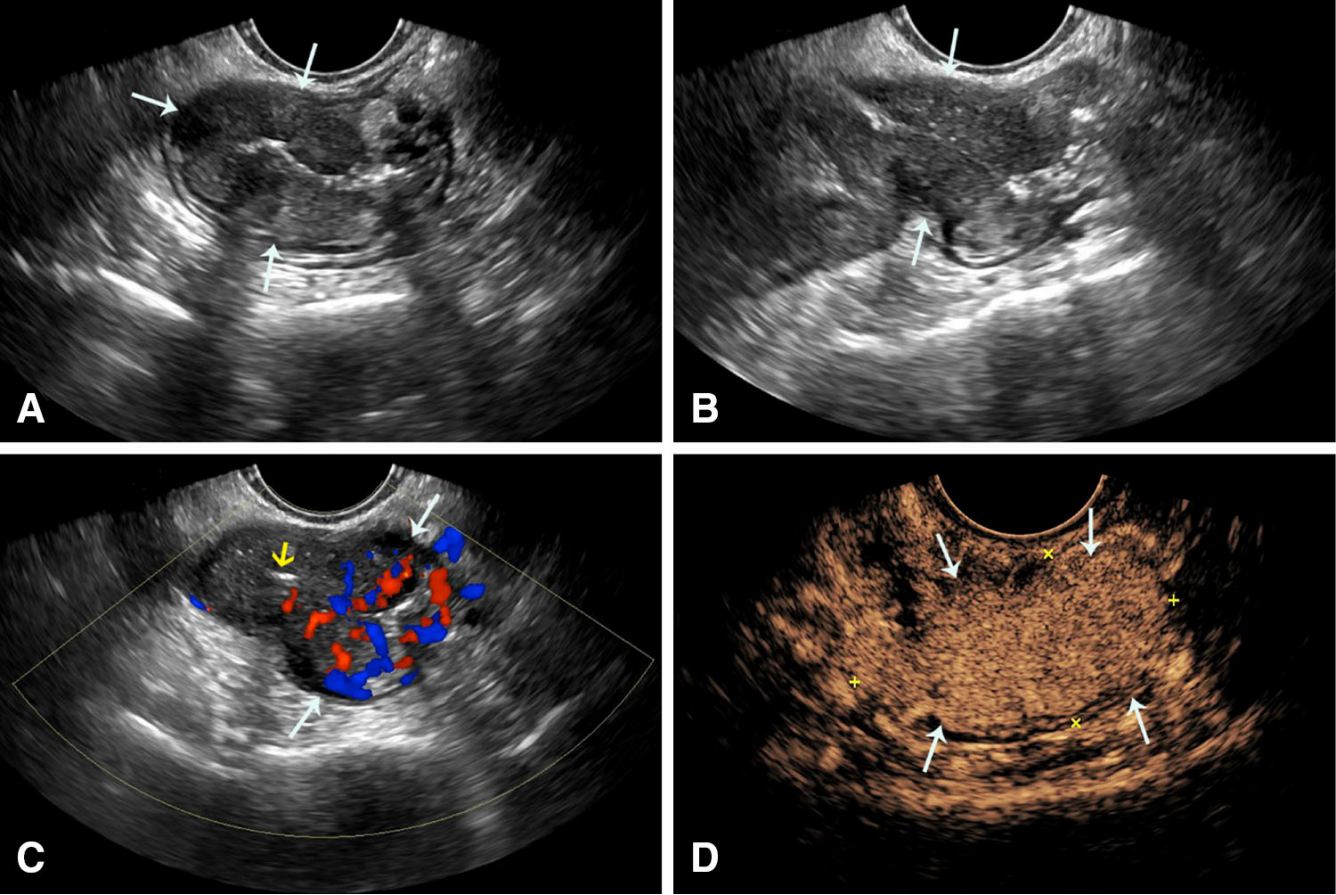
Rectal cancer on Endovaginal scan (EV) on a 37-year-old patient with rectal bleeding. A EV grayscale shows a lobulated hypoechoic asymmetric wall thickening/mass of the rectum (arrows). B Sagittal grayscale of the same abnormal segment shows an apple-core appearance (arrows). The lumen of the rectum shows as a white line on both figures A, B, and C (yellow arrow). Color Doppler shows the mass is hypervascular. D CEUS shows hyperenhancement of mass (yellow asterisk). WO is demonstrated in Supplementary Video 15
